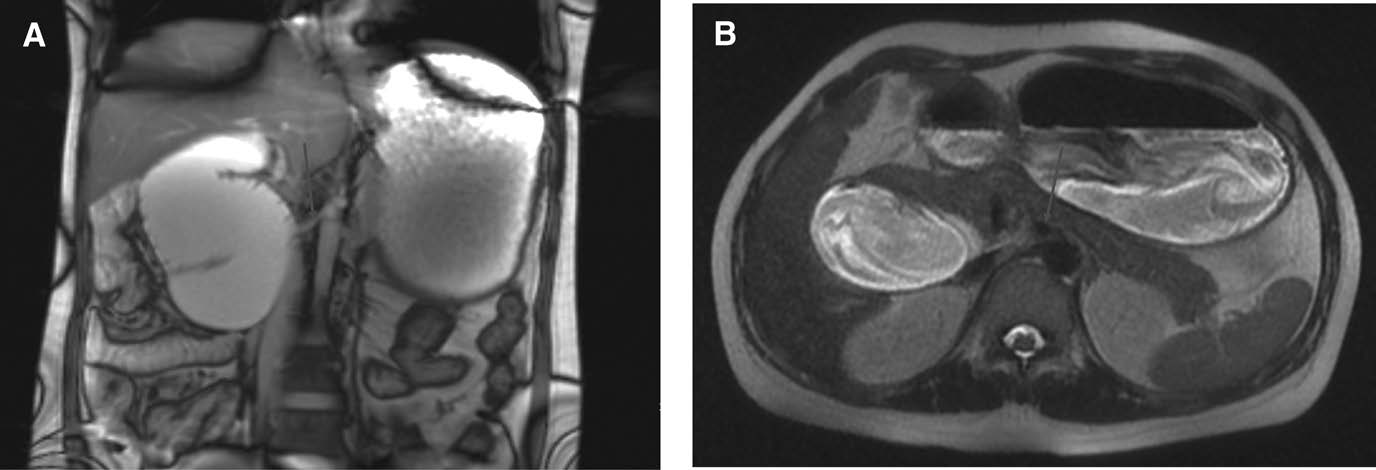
MRI image. (A). Coronal image showing the absence of a duodenal path dorsal to the SMA (purple arrow). (B) Axial image showing obvious dilation in the proximal part of duodenum and gastric cavity, and the absence of a duodenal path dorsal to the SMA (purple arrow). MRI = magnetic resonance imaging, SMA = superior mesenteric artery.
